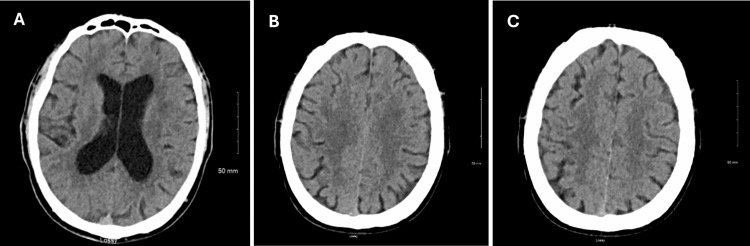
CT scan showing nonspecific changes for evaluation of altered mental status CT: computed tomography
